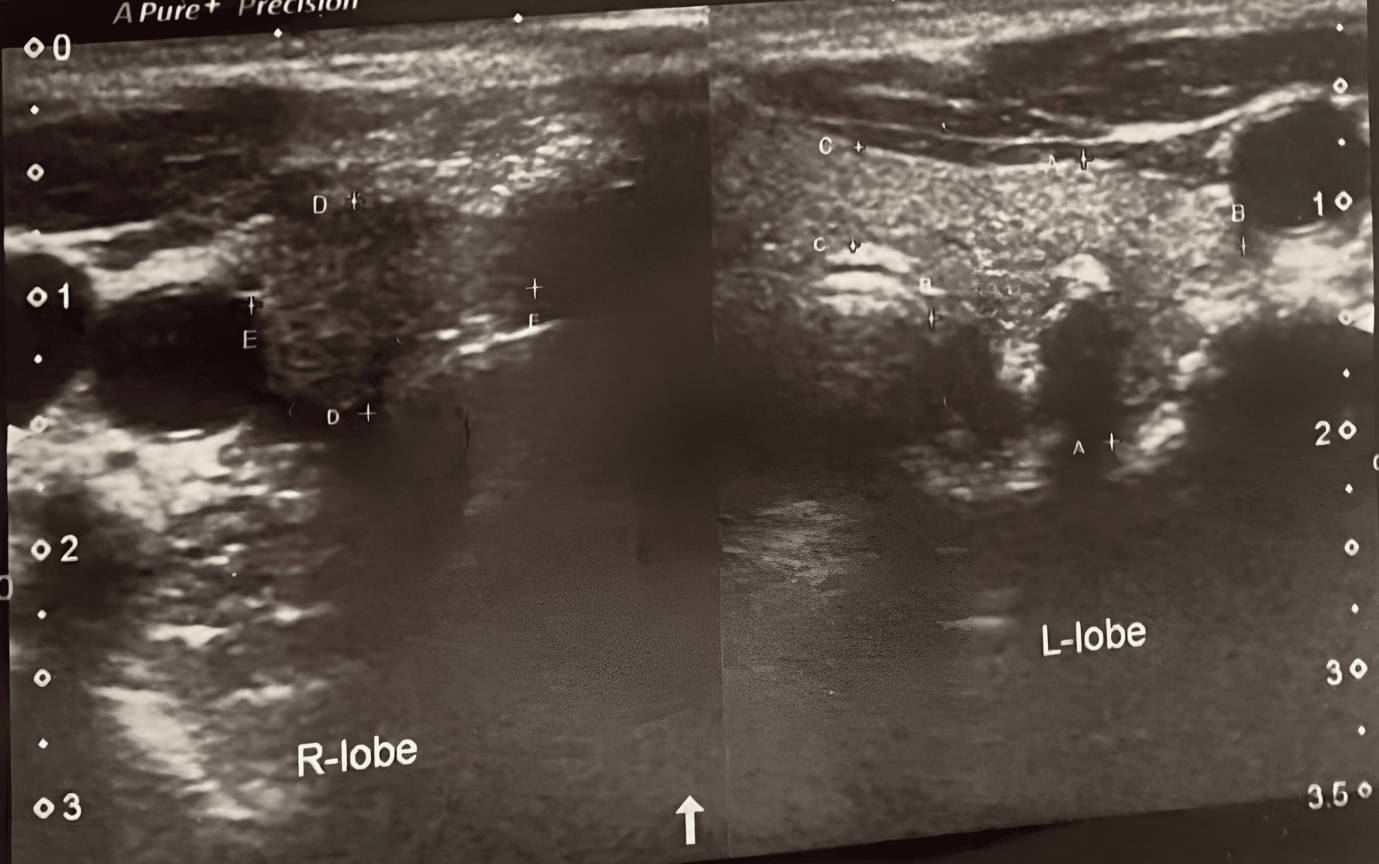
Ultrasound scan revealing a hypoechoic area in the right lobe of the thyroid gland, causing distortion of its margins extending up to the isthmus
